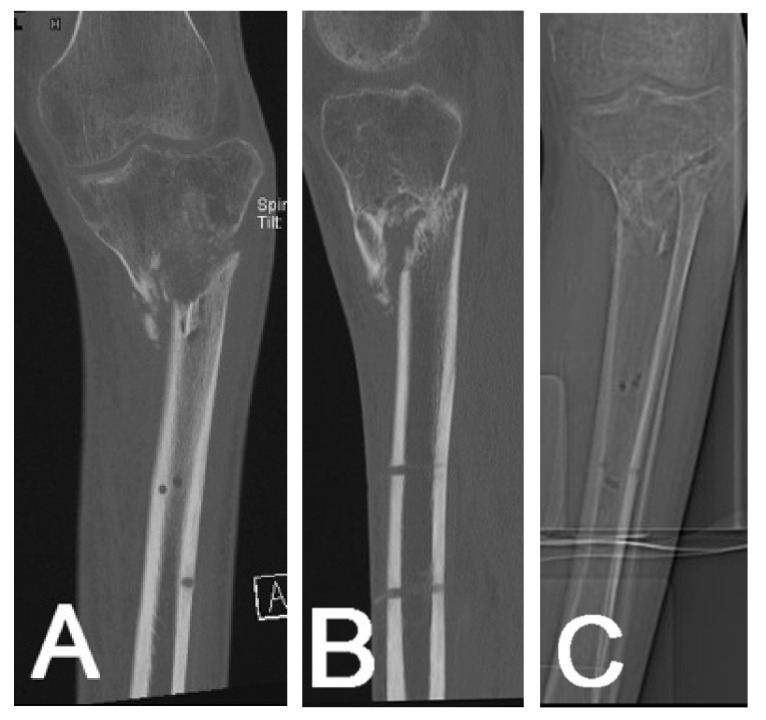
Conventional CT scan to plan deformity correction in a displaced proximal tibial fracture—2D AP view (A) and lateral view (B) showing translation and varus deformity. Scanogram from the same patient in AP view (C).
